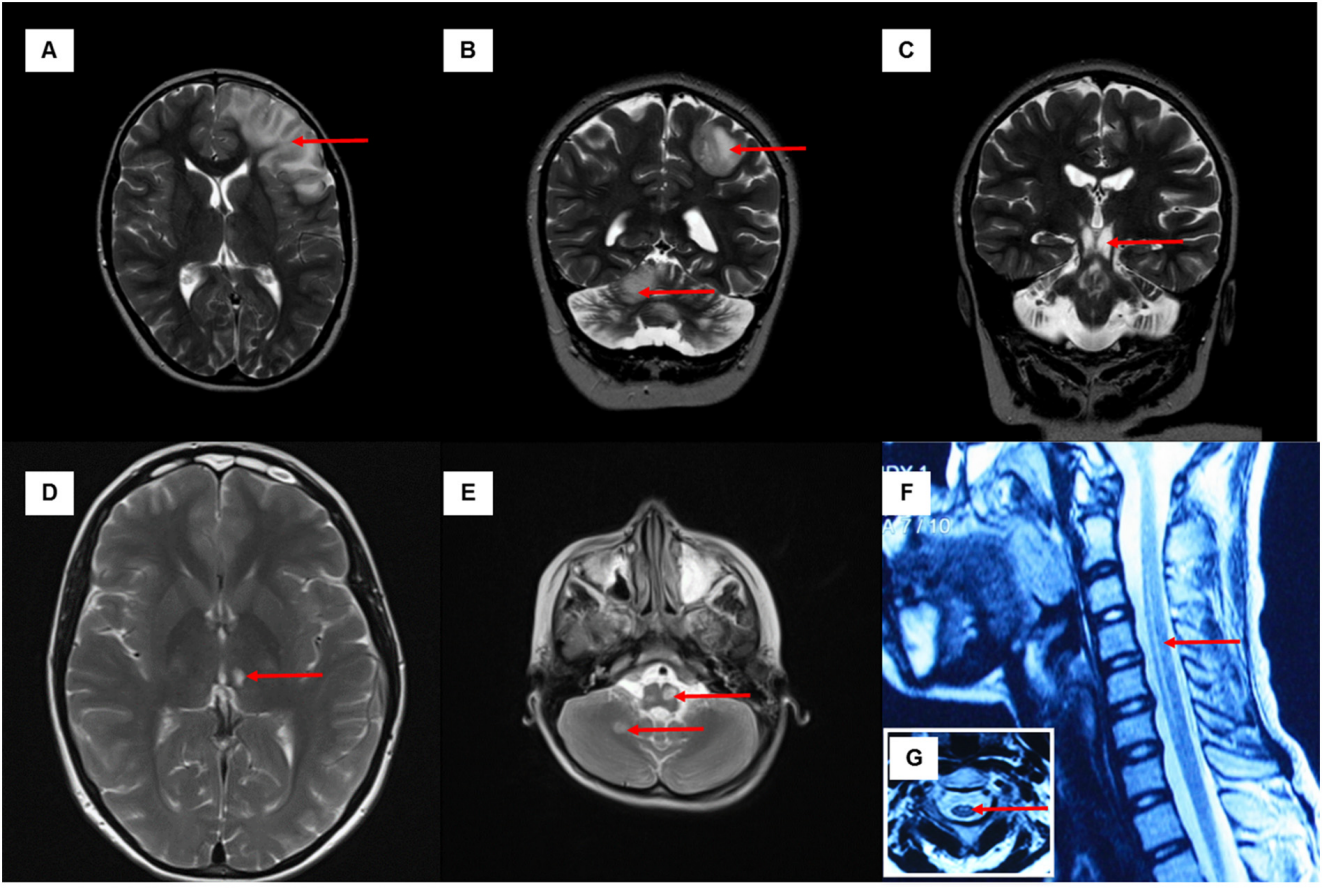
Cranial and spine MRI. Axial T2-weighted view of Patient 1·2 showed hyperintensities involving the cortical and subcortical areas of left frontal lobe when she presented with the first stroke-like episode (A); the cranial MRI performed during the subsequent stroke-like episode showed hyperintensities involving the left parietal lobe and right cerebellar cortex, suggestive of cross cerebellar diaschisis (B) and extensive signal abnormalities in the brainstem (C, coronal view). Axial T2-weighted view of Patient 2 showed an isolated, left thalamic lesion (D). Axial T2 view (E) showed a discrete signal abnormality in the right cerebellum, and asymmetrical hyperintensities in the medulla in Patient 4. The sagittal T2 view (F) showed an anterior, long hyperintensity in the cervical cord spanning C2-6 levels in Patient 5, corroborated with the signal abnormalities shown in the axial view (G).
